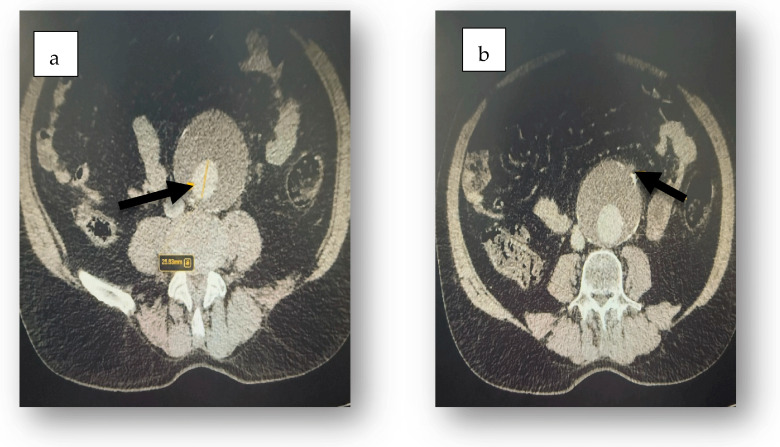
CT scan shows parietal thrombosis (black arrow) with a 2.7 cm circulating lumen (a) and thrombosis of the inferior mesenteric artery (black arrow) causing ischemic colitis (b).
